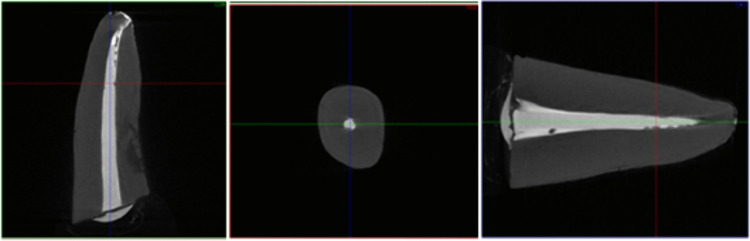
3D micro-CT images of the obturation in a sample from the TotalFill sealer without ultrasonic activation (non-UA) group. (5A) Sagittal view. (5B) Axial view. (5C) Coronal view.
